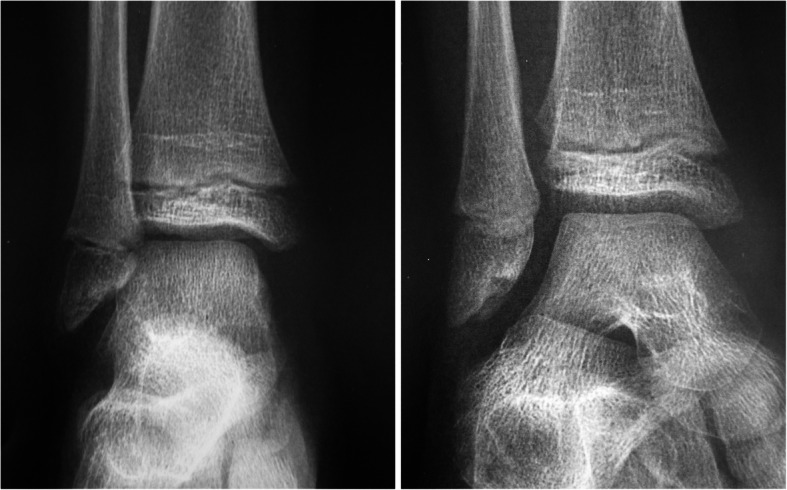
Anteroposterior and oblique x-rays prior to surgery. The non united fragment is visible at the apex of the lateral malleolus. The rest of the ankle joint is normal
